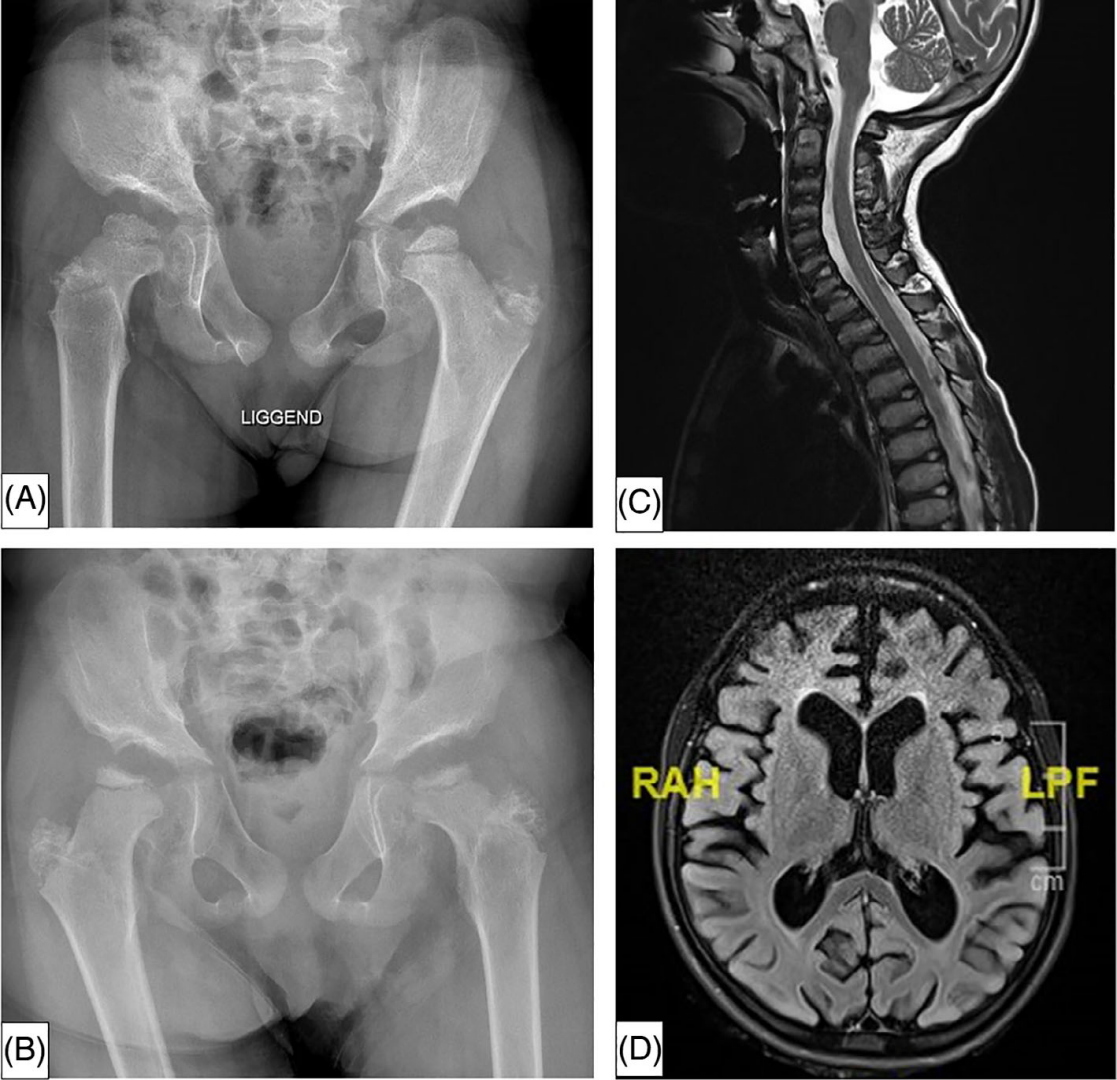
Patient 1 showed ilium hypoplasia, delay of femoral epiphyseal nuclei ossification and coxa valga on X‐ray, A, at the age of 7 years. Epiphyseal dysplasia and flattened femoral heads were apparent on X‐ray at the age of 10 years old, B, MRI studies showed dysplastic cervical vertebral bodies and platyspondyly, C, at the age of 9 years, and enlargement of the ventricular system and extensive cortical atrophy at 13 years old, D
